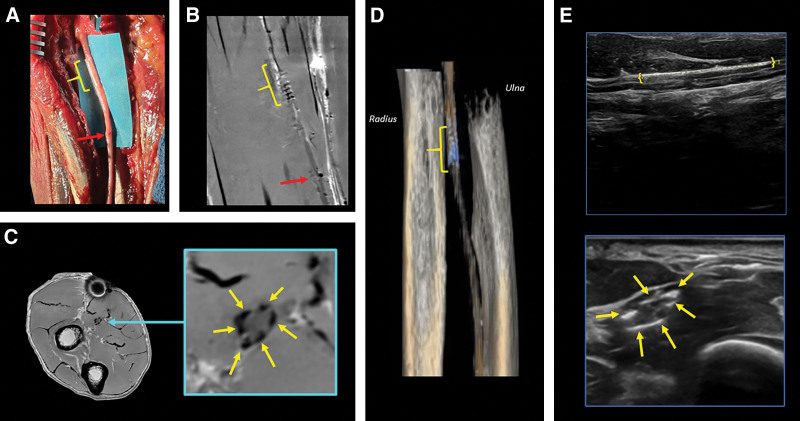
Median nerve in the cadaveric proximal forearm. A, Surgical implantation of NT (NT_4_, yellow bracket) at a transection site, and suture repair at a different transection site (red arrow). B, A coronal proton density MR image slice of the nerve longitudinally demonstrates signal void patterns from NT_4_ microhooks and the proximal nerve transection site due to the discontinuous nerve appearance (red arrow). C, Axial MRI depicts signal void patterns of the microhooks around the median nerve (yellow arrows, magnified view). D, Volume rendering of the radius, ulna, and NT_4_ (blue) around the transection site (bracket) from 3D ZTE MRI and the nerve segmentation from coronal proton density images. E, Ultrasound images. Top: longitudinal view of the median nerve and length of NT (yellow brackets). Bottom: axial view with echogenic foci demonstrating the position of the microhooks around the median nerve.
